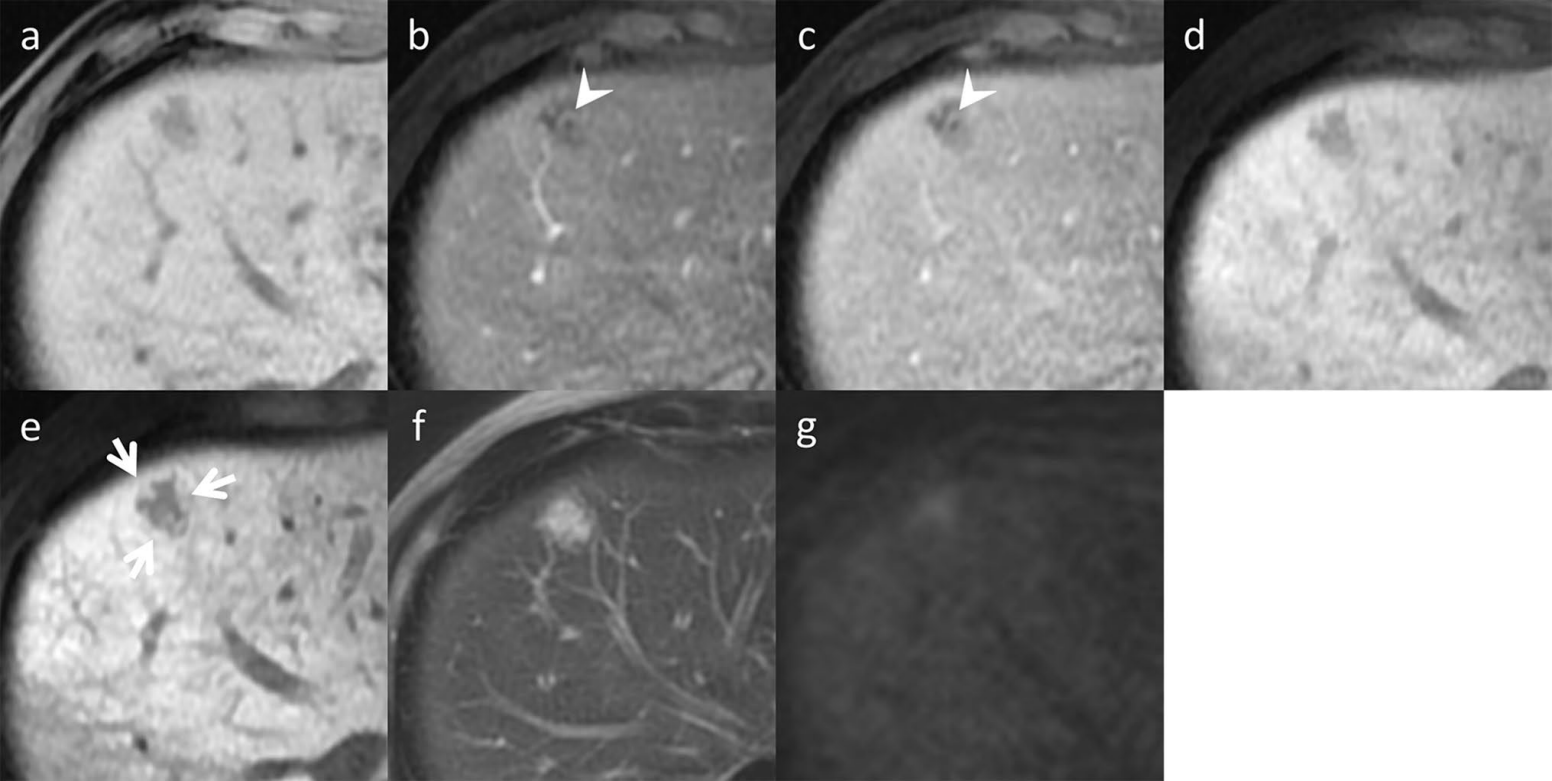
Sinusoidal obstruction syndrome (SOS) in an asymptomatic 49-year-old man, who was undergoing chemotherapy, including oxaliplatin for colon cancer. Gadoxetic acid-enhanced magnetic resonance imaging (MRI) (a pre-contrast, b arterial phase, c portal venous phase, d transitional phase, and e hepatobiliary phase) demonstrates a hypo-vascular lesion at S4/8, which demonstrates hyper-intensity on f. T2-weighted imaging and g. diffusion-weighted imaging with b value 800 s/mm^2^. The apparent diffusion coefficient value of this lesion is not reduced (data not shown). Peripheral gadoxetic acid uptake is observed in the hepatobiliary phase (arrows) and intra-lesional vessels in the arterial phase as well as the portal venous phase (vessel-penetrating sign, arrowheads). These are key imaging findings that differentiate mass-forming SOS from liver metastases
